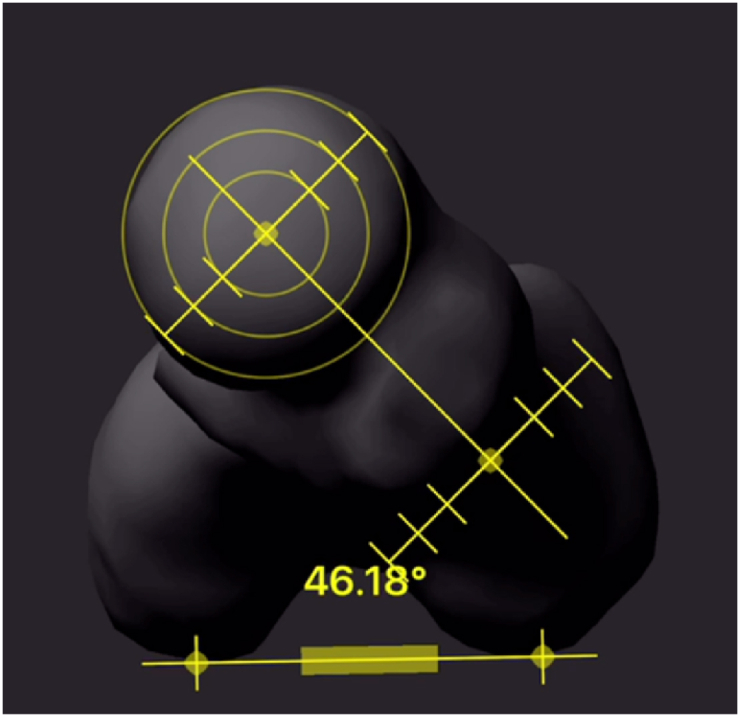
Sample image of the 3D reconstruction from two plane X-rays and the angle measurement using FEMORA® software.
